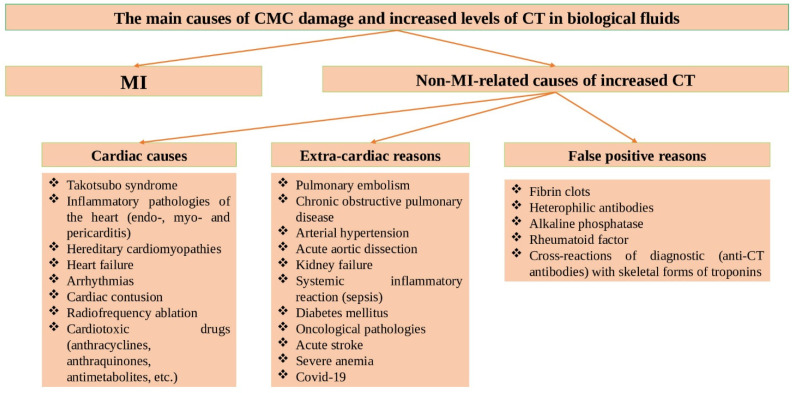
Main reasons of CMCs damage and increased CTs levels in body fluids. List of abbreviations. CT—cardiac troponins; MI—myocardial infarction, CMC—cardiomyocytes.
